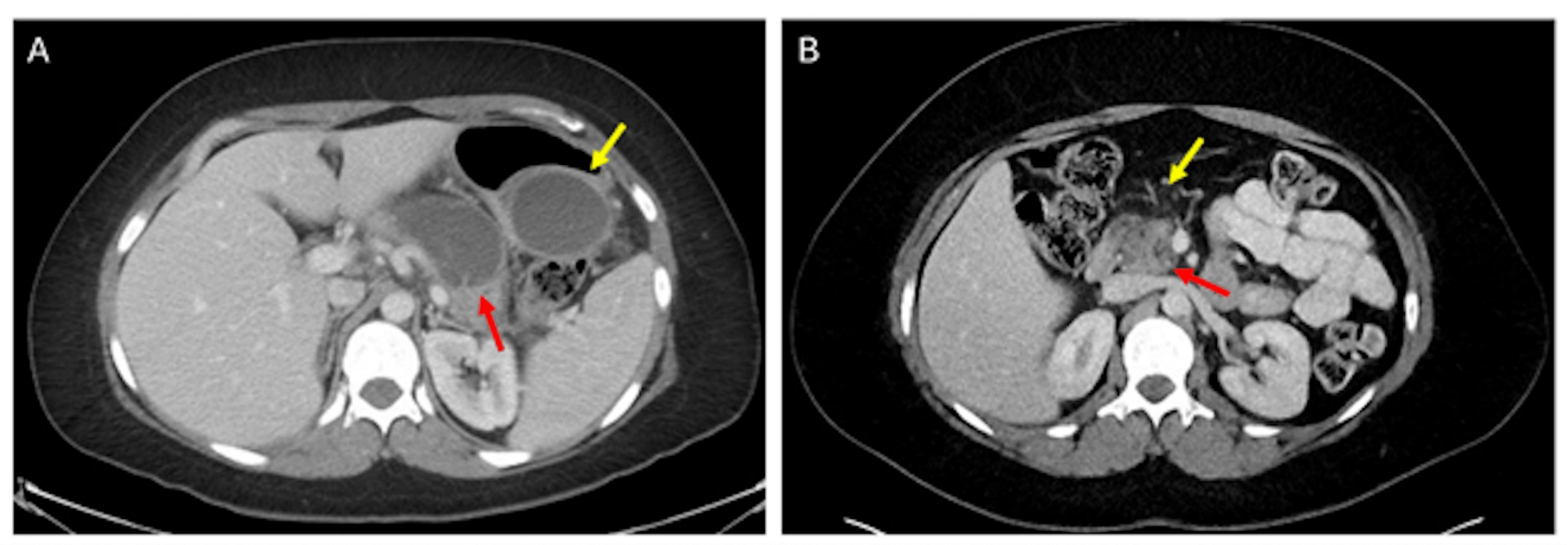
CT images of pancreatic cyst evolution Axial CT scan of the abdomen with IV contrast obtained during the patient's first episode of pancreatitis (A) demonstrates fluid-attenuating lesions within the body of the pancreas (red arrow) and along the gastric wall (yellow arrow), which both represent pseudocysts in the setting of recent pancreatitis and demonstrate the patient's complicated course. Axial CT scan of the abdomen with IV contrast from two years later (B) demonstrates pancreatic head edema (red arrow) and surrounding fat stranding (yellow arrow), consistent with a repeat episode of acute pancreatitis. CT: computed tomography
